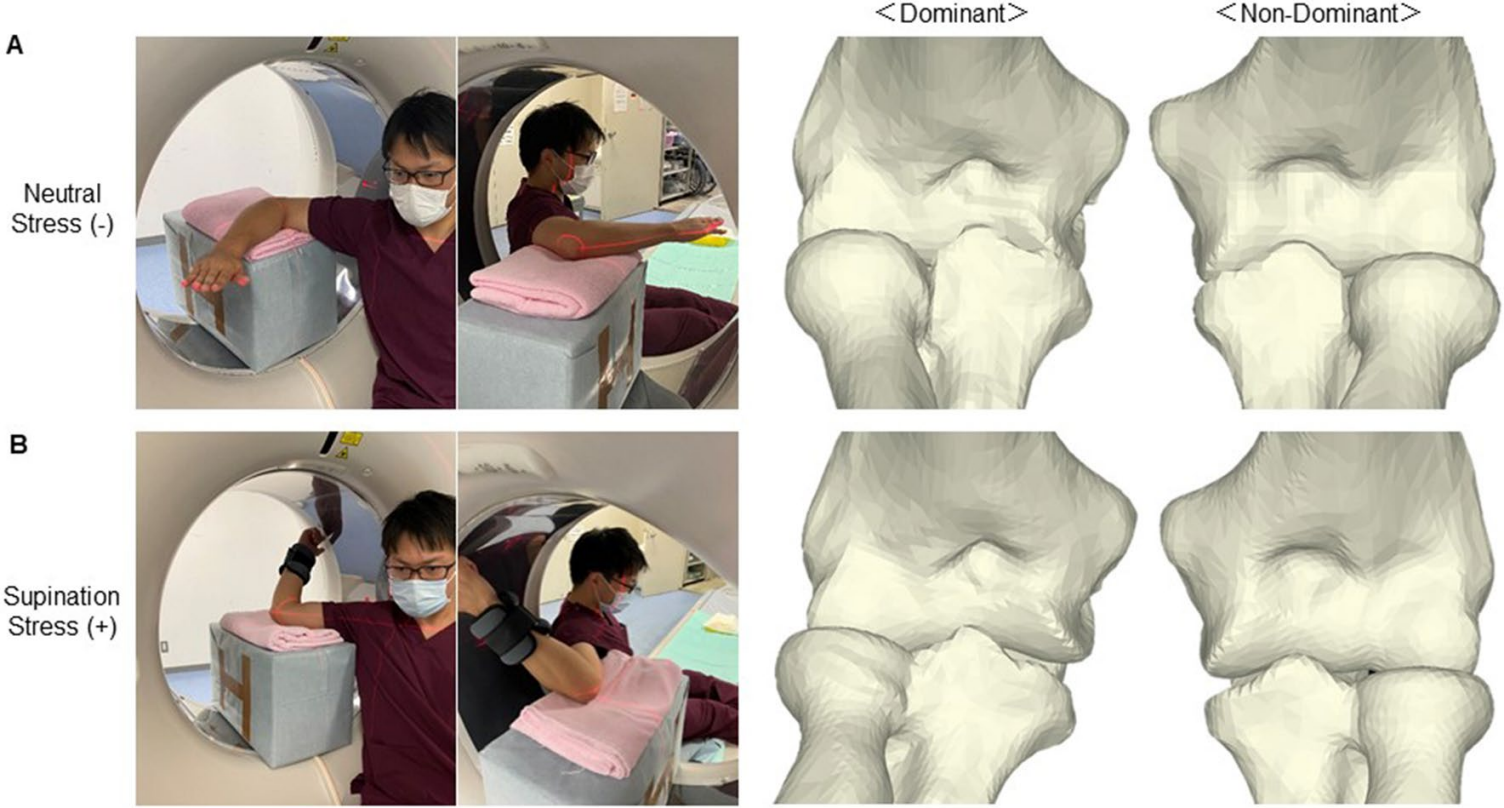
Acquisition of 3D bone models. (A) A CT image is obtained with the shoulder in abduction of 90° and the elbow flexed at 90° without valgus stress. 3D bone models of the humerus, radius, and ulna surfaces are created from each arm position. (B) With elbow valgus stress.
